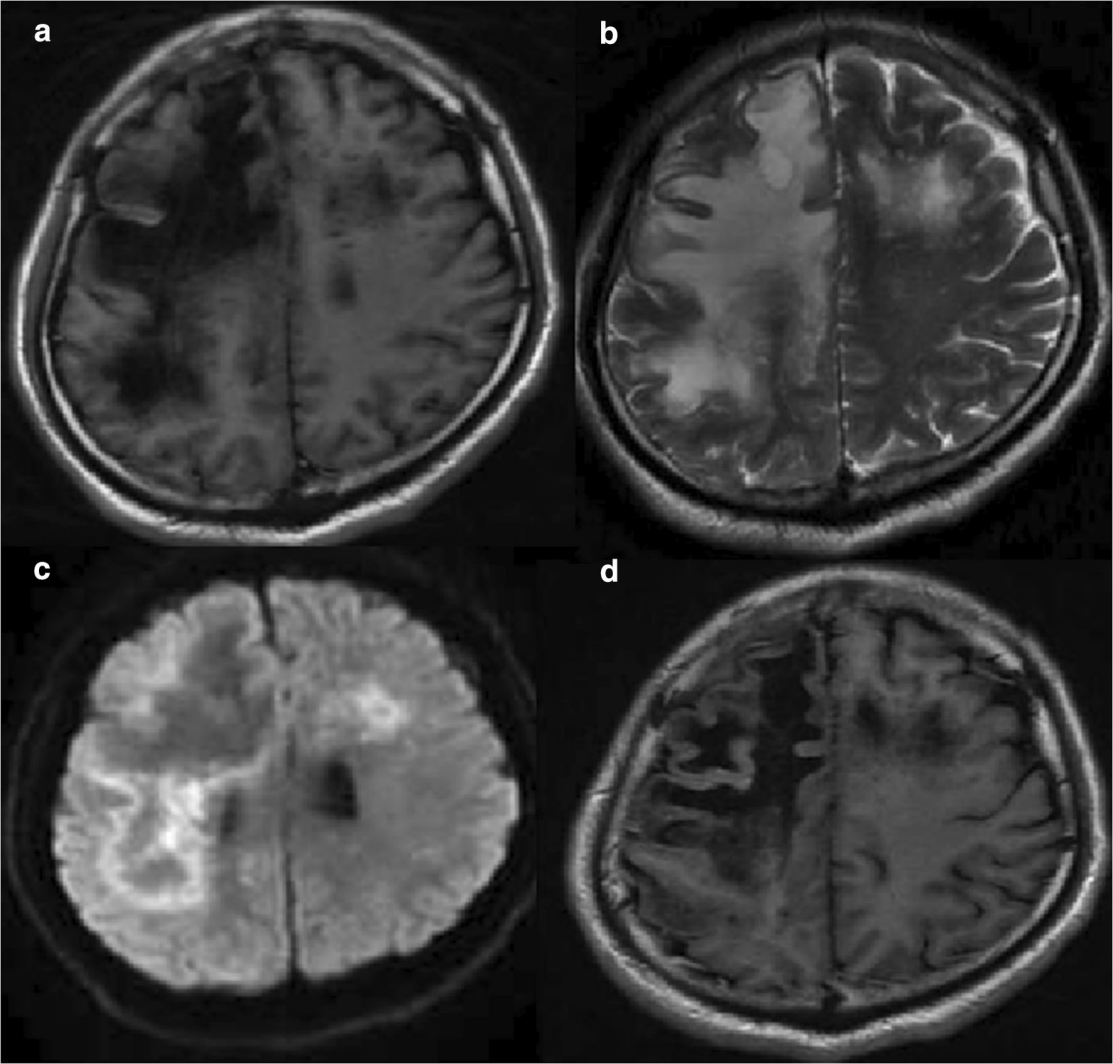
After 2 weeks of treatment, MRI of intracranial lesions got worse (a, b, c, d). The lesions were hypointense (dark) on T1 and hyperintense (white) in T2 sequences, showing no enhancement. DWI demonstrated ring-shaped marginal and small patch-like high signal shadows
